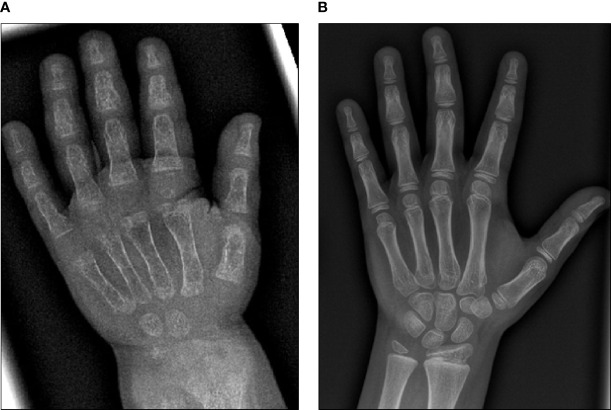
X-ray images of the left hand before and after 42 months of burosumab treatment. (A) X-ray image of the left hand at 3.5 years of age. Note gracile, undermineralized bone structure with loss of definition of the provisional calcification zone at the epiphyseal/metaphyseal interface and disorganization of the growth plate. Bone age was determined to be 1 year. (B) Follow-up X-ray image of the left hand at 8 years of age. According to the RGI-C score, a substantial improvement in ricket severity (RGI-C score +2) is noted. Overall skeletal maturity with a nearly age-appropriate bone age of 7.5 years was observed.
